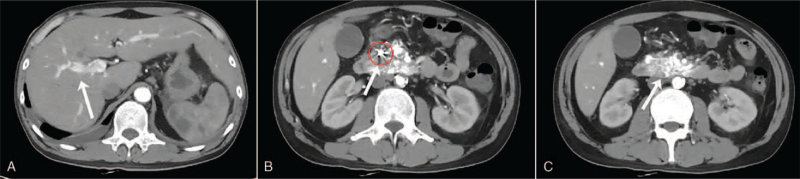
Enhanced abdominal CT findings. Enhanced abdominal CT indicating that the portal vein was filled early with arterial blood during the arterial phase (A), and dot-like small vessels with intense signals in the lesion of the pancreatic head were observed (B). A coil-like metal shadow was seen in a branching vessel of the gastroduodenal artery (read cycle) (C).
